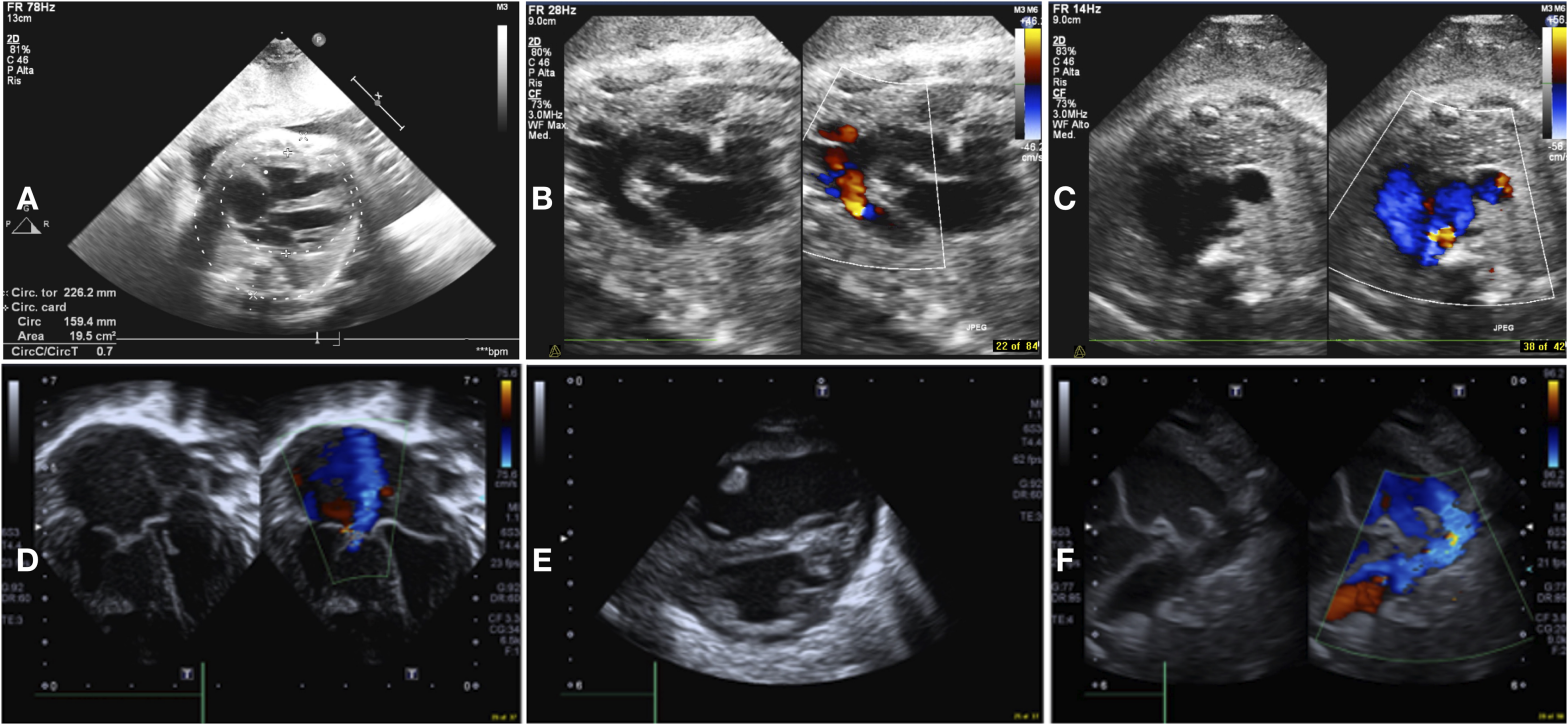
Fetal and neonatal echocardiographic images. Fetal images: (A) increased cardiothoracic index, 4-chamber axial view; (B) retrograde flow in aortic arch, long axis view; (C) superior vena cava dilation, 3 vessels view. Neonatal images: (D) right heart dilatation and tricuspid regurgitation, apical 4 chamber view; (E) flattening of the interventricular septum, parasternal short axis view; (F) right to left shunt at the level of the ductus arteriosus, standard “three-finger” ductal view.
